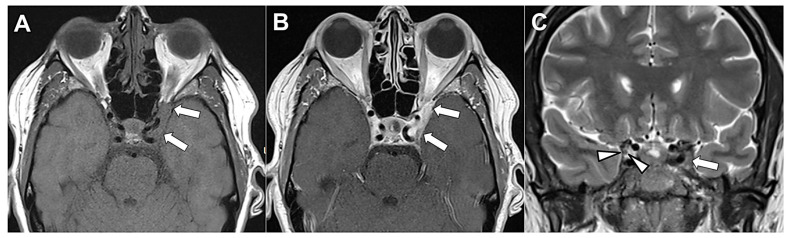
MRI findings in left-sided Tolosa–Hunt syndrome with oculomotor nerve (OMN) involvement. (A) Axial T1-weighted non-fat-saturated image demonstrates hypointense soft tissue replacing and mildly expanding the left cavernous sinus, extending toward the superior orbital fissure (thick arrows). (B) Axial T1-weighted post-contrast non-fat-saturated image shows homogeneous abnormal enhancement of this soft tissue (thick arrows). (C) Coronal T2-weighted image shows the lesion as mildly hypointense, with associated mild expansion of the left cavernous sinus (thick arrow). Note how the right OMN is clearly visualized within the right normal cavernous sinus (arrowheads), whereas the left OMN cannot be seen due to the surrounding abnormal tissue. Clinically, the patient had presented with acute unilateral periorbital pain, ipsilateral ptosis, diplopia, and impaired adduction, elevation, and depression of the left eye. Therefore, overall, the imaging findings were consistent with Tolosa–Hunt syndrome, an idiopathic granulomatous inflammatory process involving the cavernous sinus and causing OMN palsy secondary to cavernous sinus inflammation. Image Credit: Dr. Nikolaos-Achilleas Arkoudis and Prof. Georgios Velonakis.
